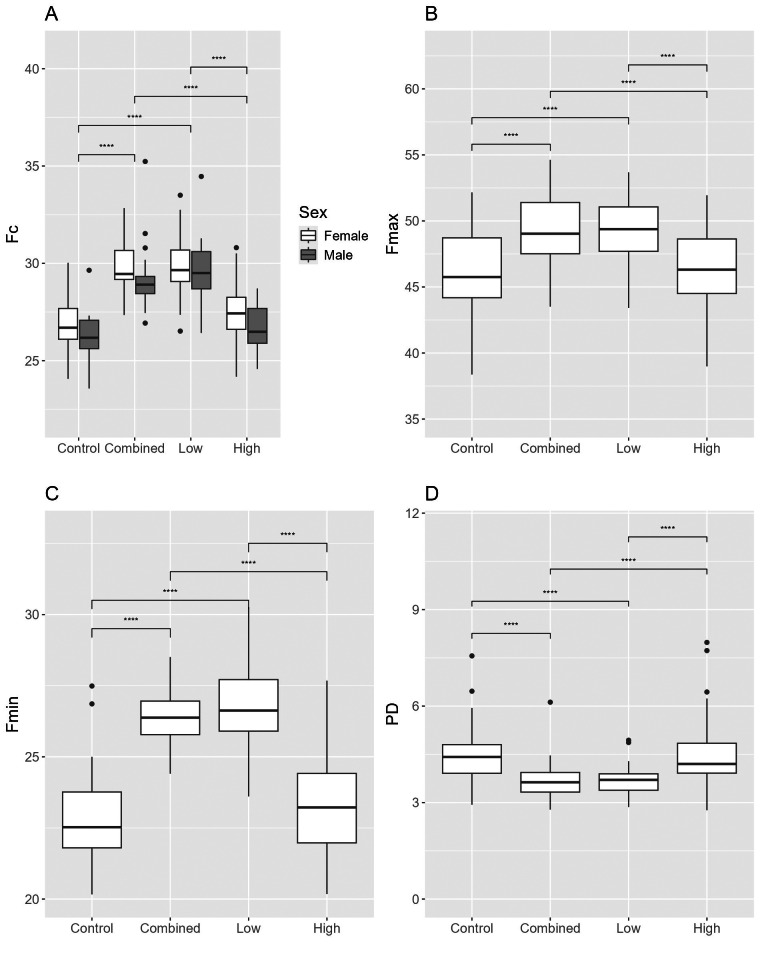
Differences in echolocation characteristics of Brazilian free-tailed bats (*Tadaria brasilienses*) during ultrasonic deterrent trials. Asterisks indicate significant differences (* = 0.05,  = 0.01, * = 0.001, and  = 0.0001). Treatments included Control and ultrasonic deterrent emissions (Low (20–32 kHz), High (38–50 kHz), and Combined (20–50 kHz)). (A) characteristic frequency (Fc), (B) maximum frequency (Fmax), (C) minimum frequency (Fmin), and (D) pulse duration (PD). Significant differences are indicated above the box plots.
